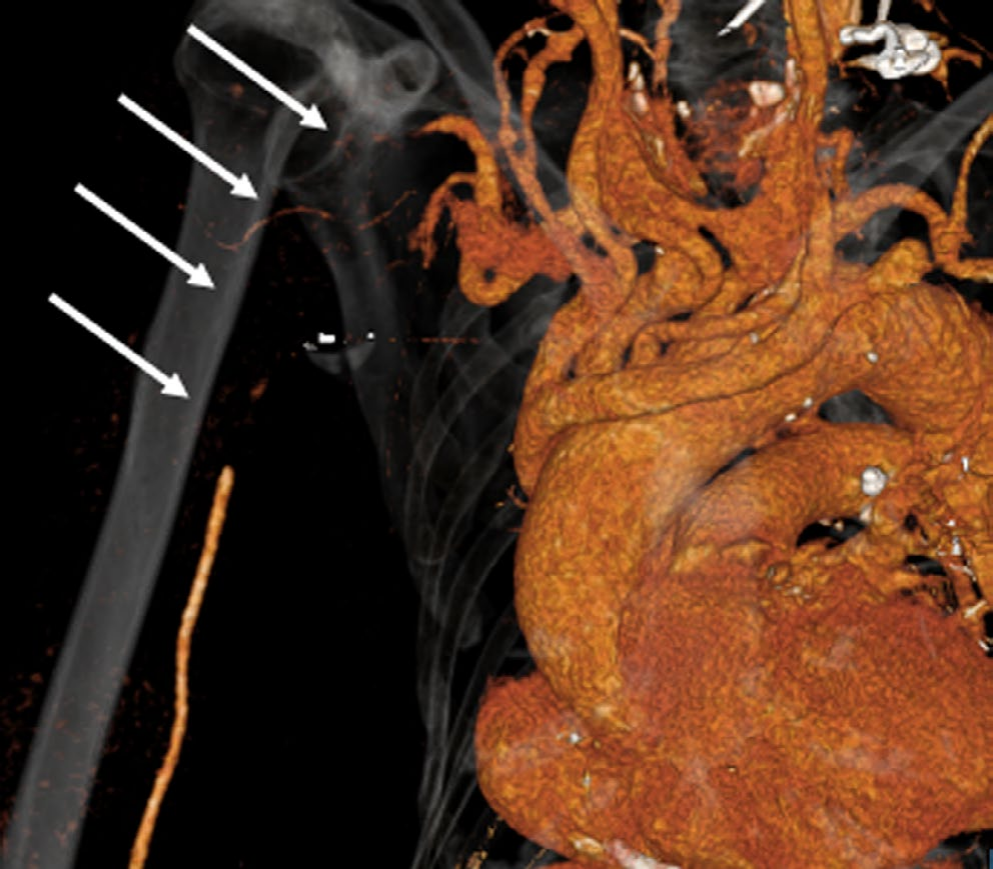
Three‐dimensional computed tomographic angiography revealed an occlusion lesion from the axillary artery to the brachial artery
